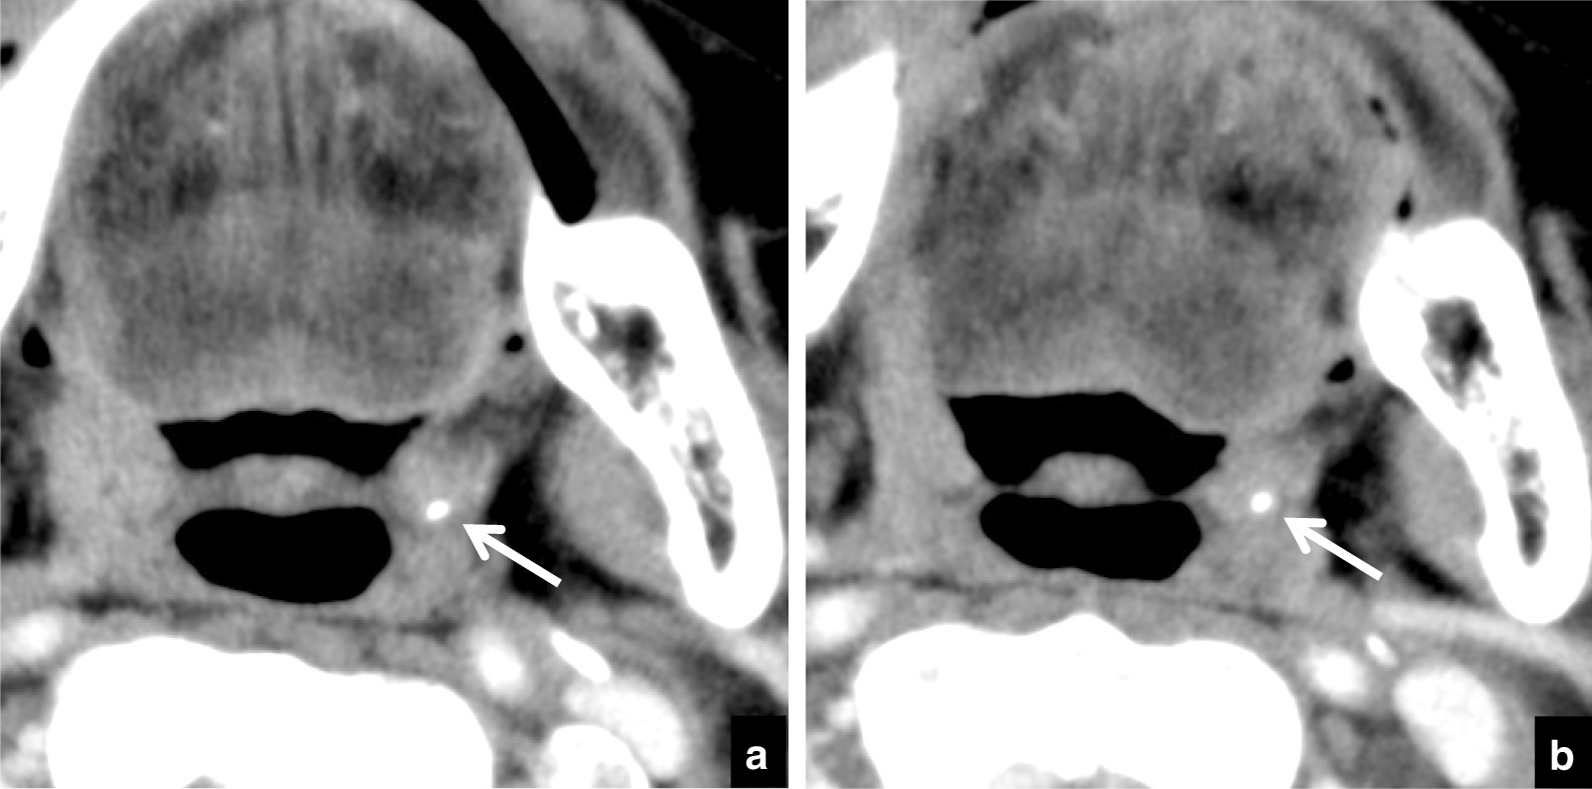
A 70-year-old subject with malignant tumor of the left mandibular gingiva in whom tonsillolith size showed no dynamic change on CT. a Axial CT image at the palatine tonsillar level obtained at the initial examination. A tonsillolith (arrow) is seen as a high-density dot. The long axis of the tonsillolith was 2.7 mm. b Follow-up CT image at the same level 14 months later. The long axis of the tonsillolith (arrow) was 2.7 mm, the same as at the initial examination
